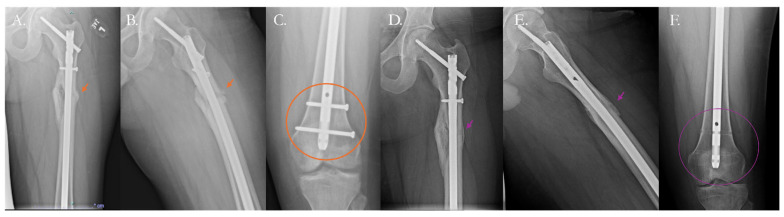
Femur nonunion with BMAC and nail dynamization. 27-year-old male with a nonunion following intramedullary nailing of a displaced comminuted femur fracture. Six months after his initial surgery the patient was diagnosed with nonunion of the fracture. (A,B) Radiographic findings of femur nonunion with residual fracture line through region of hypertrophic callus (orange arrow). (C) Distal locking screws (orange circle) in initial intramedullary nail construct. (D,E) Radiographic evidence of union at one year after nail dynamization and BMAC placement, with resolution of multicortical fracture lines (purple arrow). (F) Evidence of removed distal locking screws (purple circle) to dynamize intramedullary nail for compression of proximal fracture segment.
